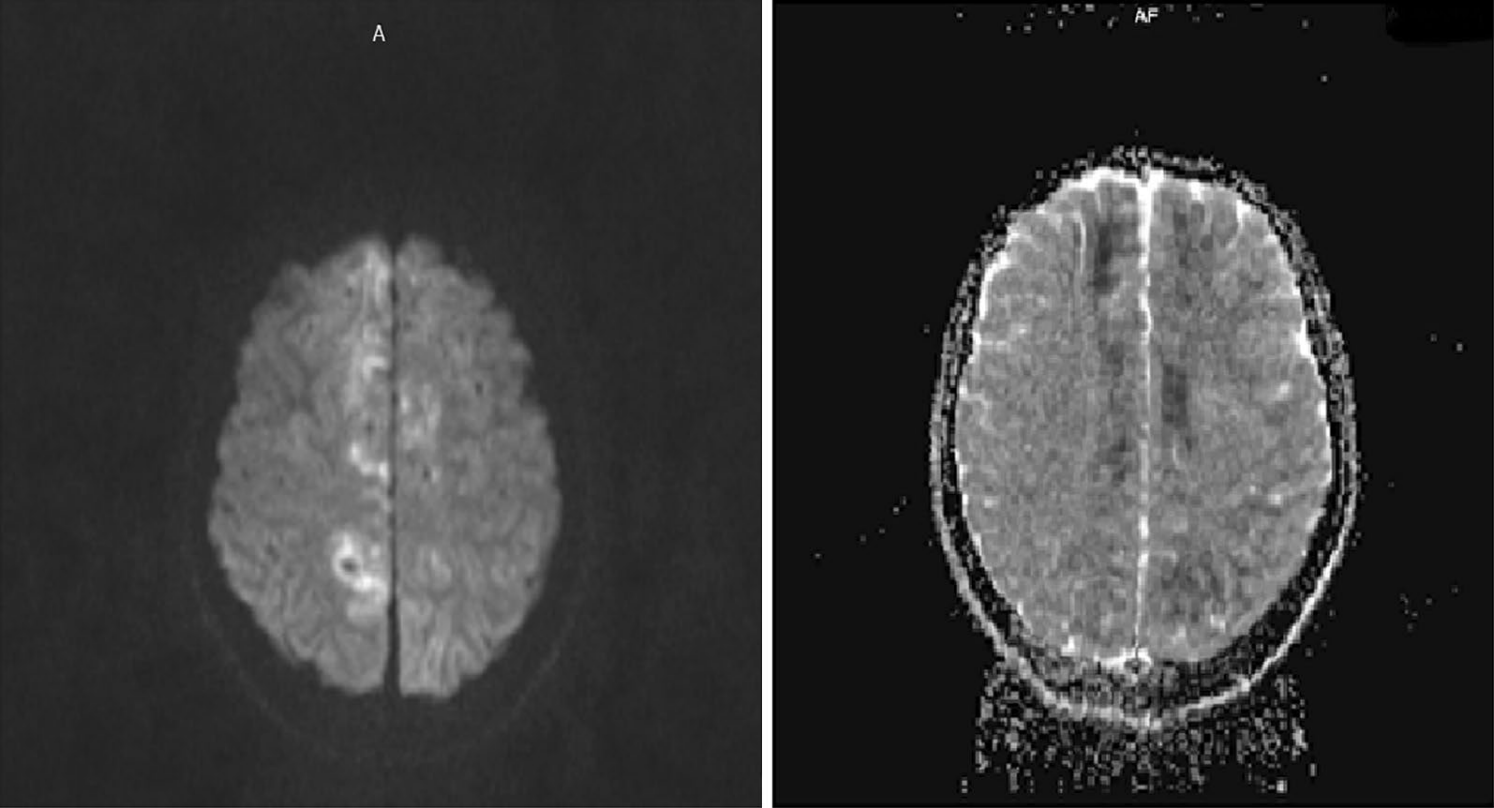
Axial diffusion weighted and ADC magnetic resonance imaging of the brain demonstrates bilateral cortical diffusion restriction in frontal, parietal and occipital lobes
